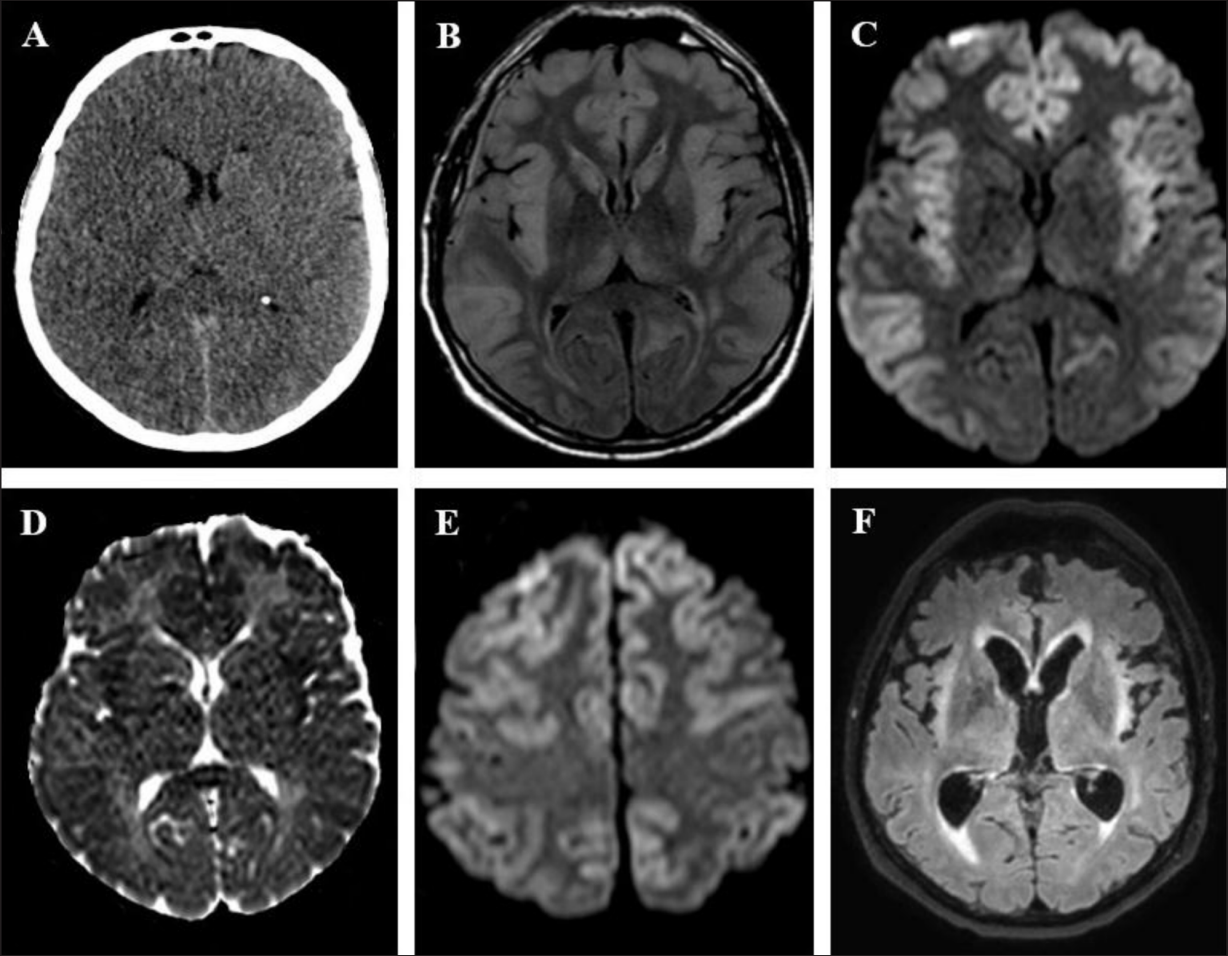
A 56-year-old man presented with status epilepticus and coma four days after bi-pulmonary graft. (A) Non-enhanced CT shows ventricular and sulcal effacement suggesting brain edema. (B) Fluid-attenuated inversion recovery (FLAIR) sequence shows bilateral cortical swelling and high signal, with sparing of the occipital cortex, as well as signal abnormalities in both caudate nuclei and thalami. (C) Diffusion weighted-imaging (DWI; b-value = 1000 s/mm^2^) and (D) apparent diffusion coefficient (ADC) map show respectively high and low signal of the cortex at the same levels, consistent with cytotoxic edema. (E) DWI sequence at a higher level shows sparing of the peri-rolandic cortex. (F) Follow-up FLAIR imaging at four weeks shows development of atrophy and gliosis in insula bilaterally.
